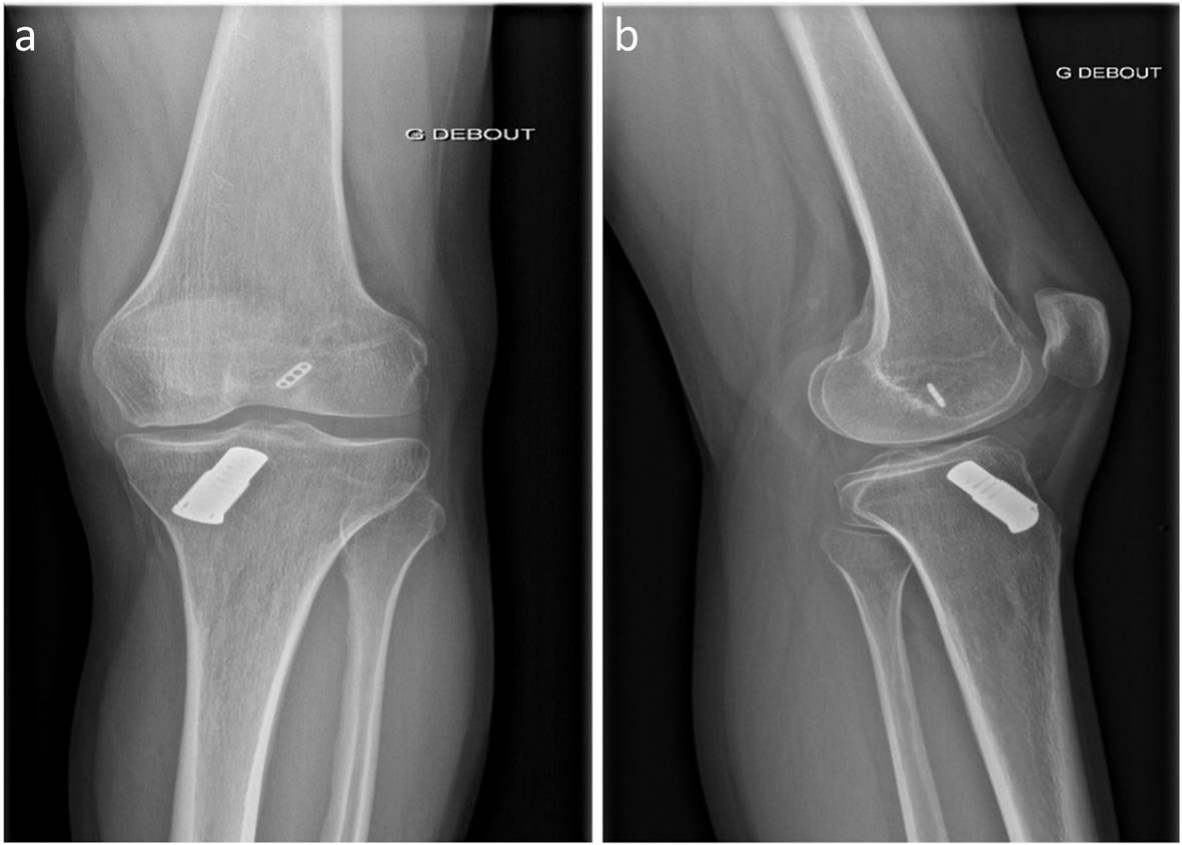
Preoperative anteroposterior (a) and lateral (b) X-rays before implant removal showing significant malposition of the femoral tunnel and improper position of the endobutton which had not flipped over the femoral cortex
